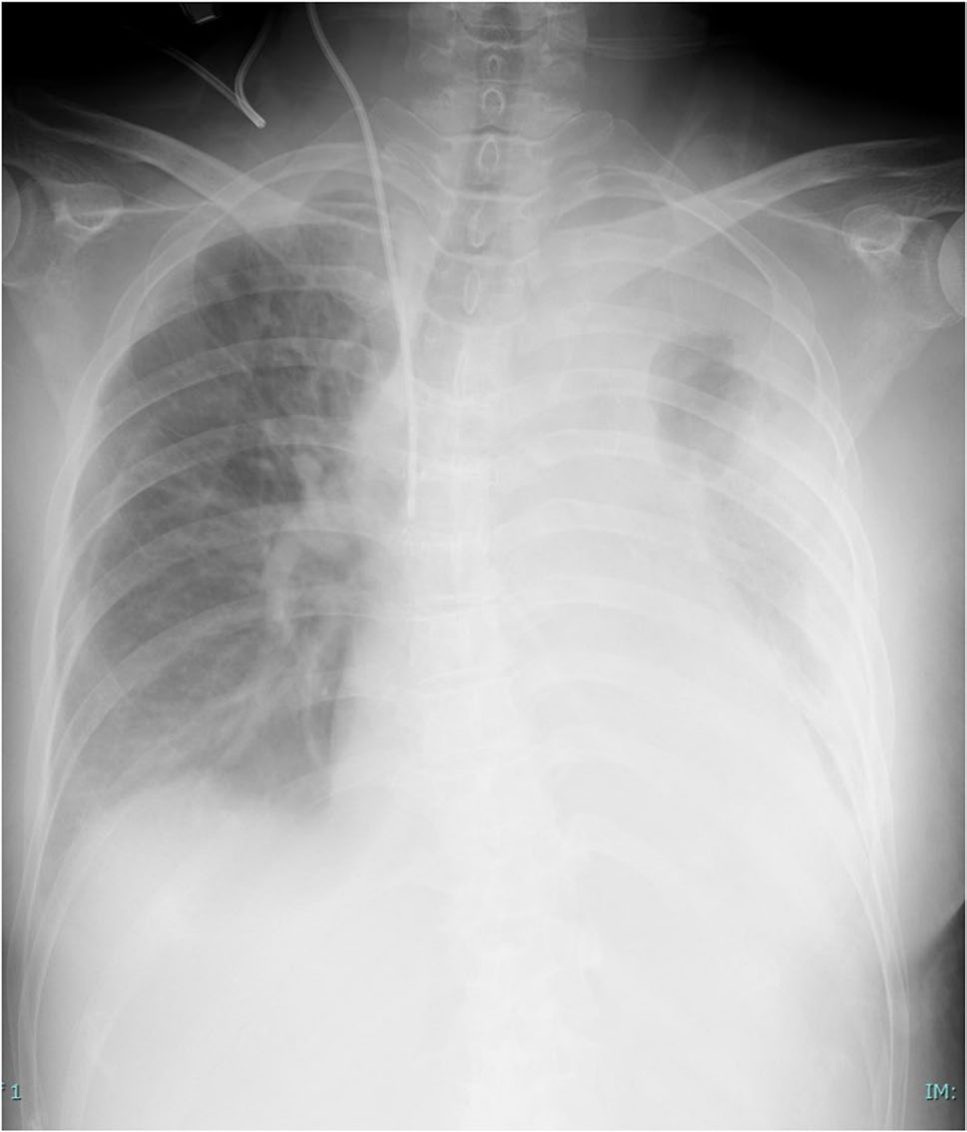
Chest X-ray on hospital day 3 shows marked exacerbation of left lung infiltration
